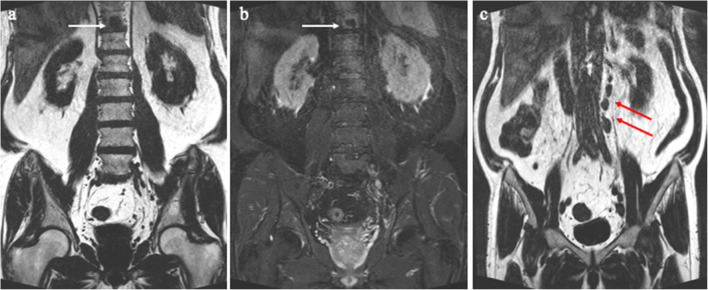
A 64-year-old patient with cT2/radT3a, PSA 39 ng/ml, and ISUP 5. The white arrow indicates a solitary metastasis in the 11^th^ thoracic vertebral body. The red arrows indicates multiple non-regional paraaortic lymph node metastases. In this case, the patient underwent a supplementary MRI of the complete column verifying an isolated bone metastasis. a Coronal T2_tse_dixon fat, b coronal T2_tse_dixon water, (c) coronal T2_tse_dixon fat
